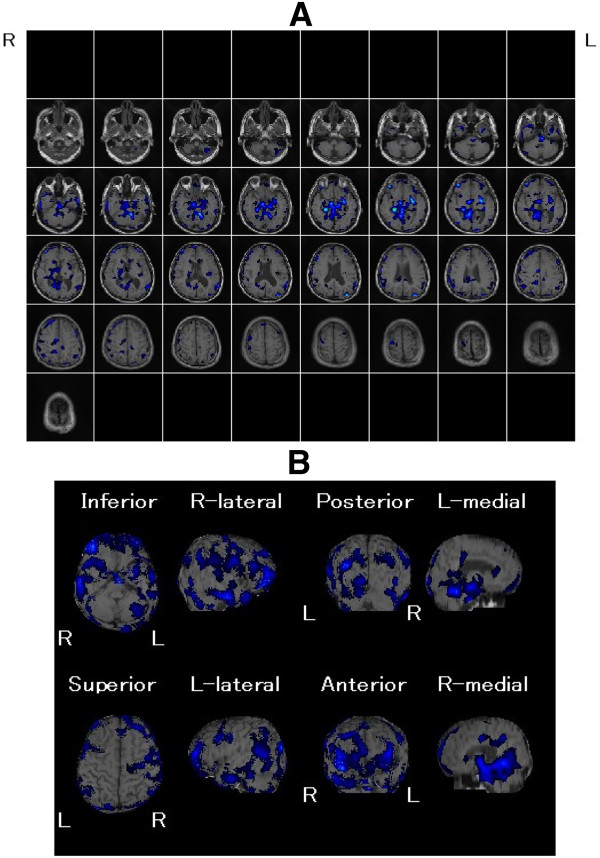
eZIS from interictal - ictal Tc-99 m ECD-SPECT (A), (B). Hypoperfusion is shown as a blue area in cingulate cortex, basal ganglia.
